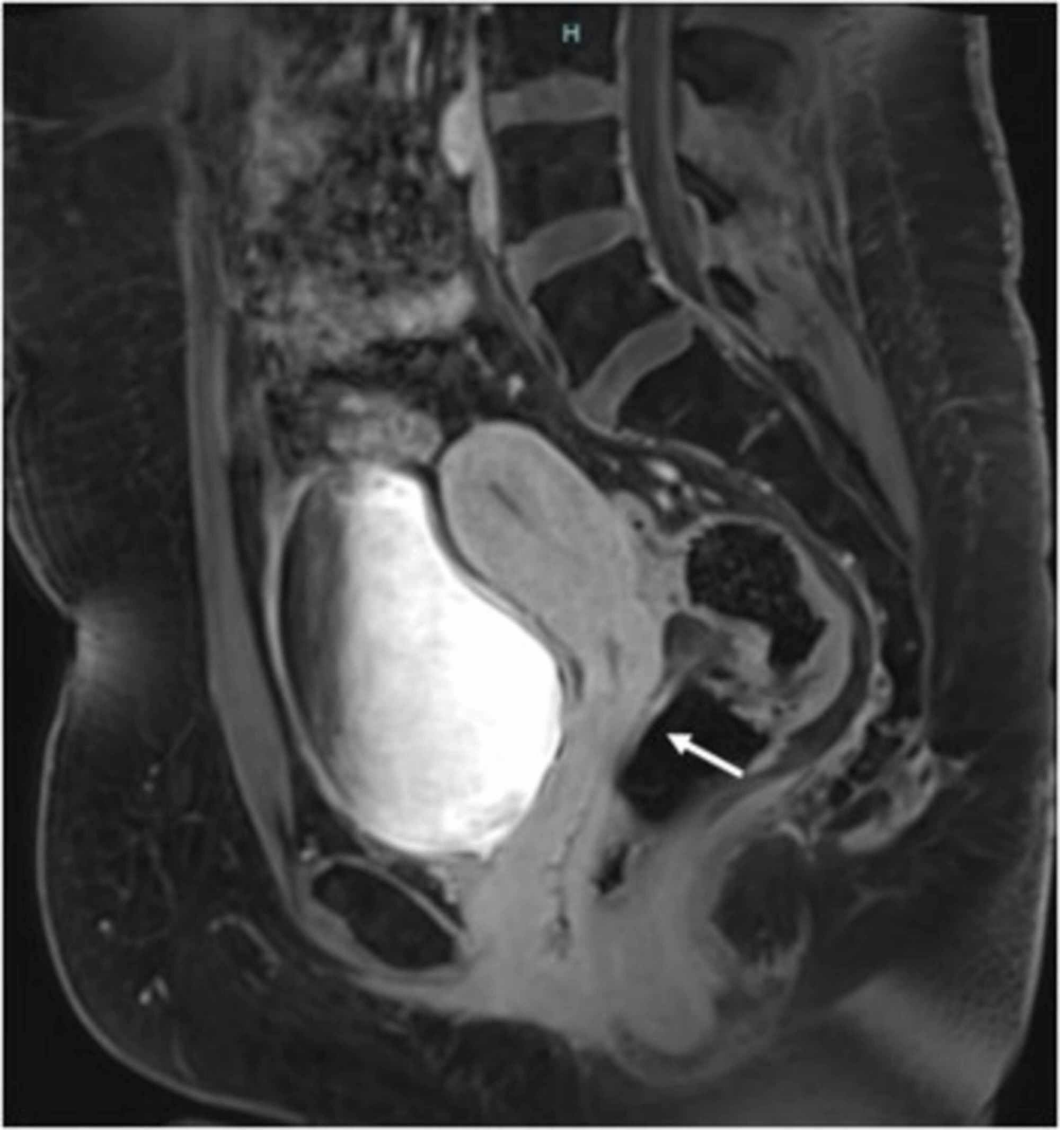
Pelvic MRI after treatment showing resolution of the cervical mass
